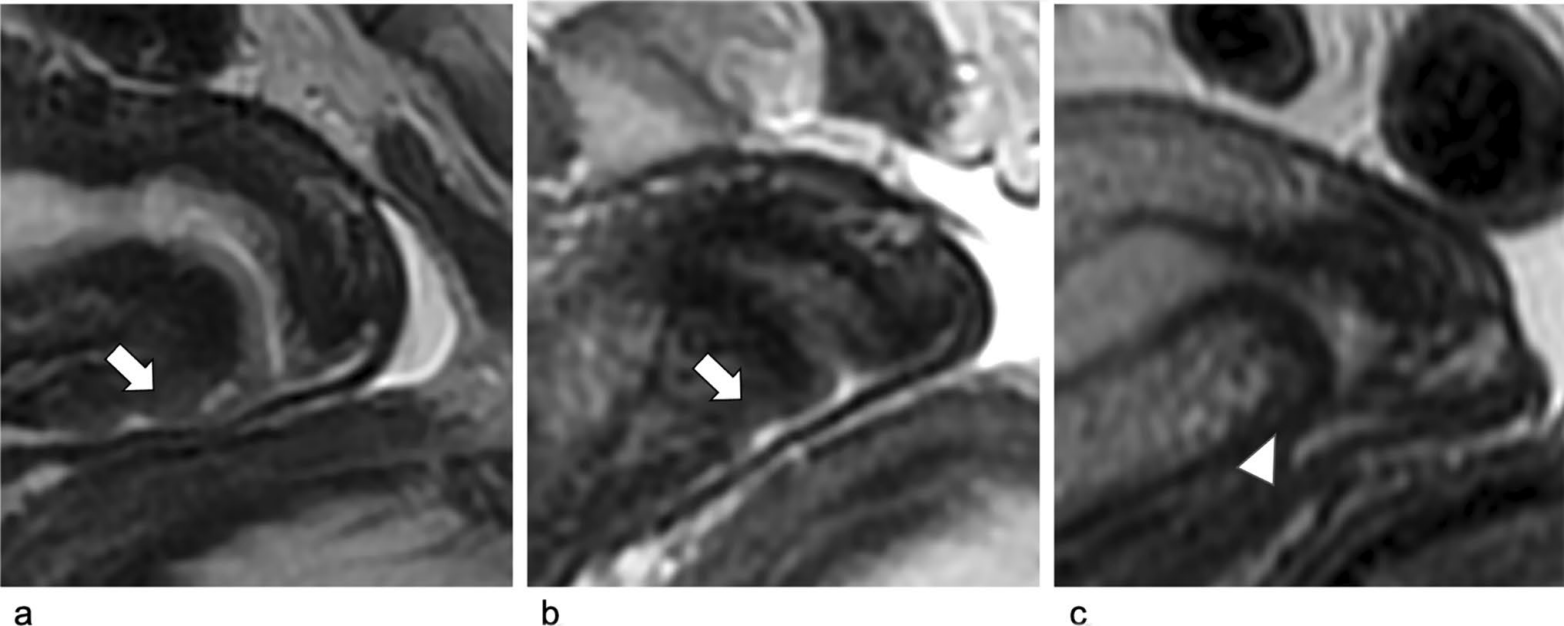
Post-conization MRI in a 29-year-old woman with cervical adenocarcinoma (FIGO staging IIA1), who underwent CKC after NACT. Sagittal T2-w images at baseline (a), after NACT (b) and after CKC (c). Baseline MRI shows the hyperintense cervical mass (arrow in a). Post-NACT shows reduction of the cervical lesion with persistence of a small hyperintense area within the anterior cervical lip (arrow in b), with diffusion restriction on DWI and ADC map (not shown). Post-conization sagittal T2-w images (c) show modfications represented by shortening of the cervical canal, and reduction of dimension of both anterior and posterior lips, better evident on the anterior lip (arrowhead in c.)
